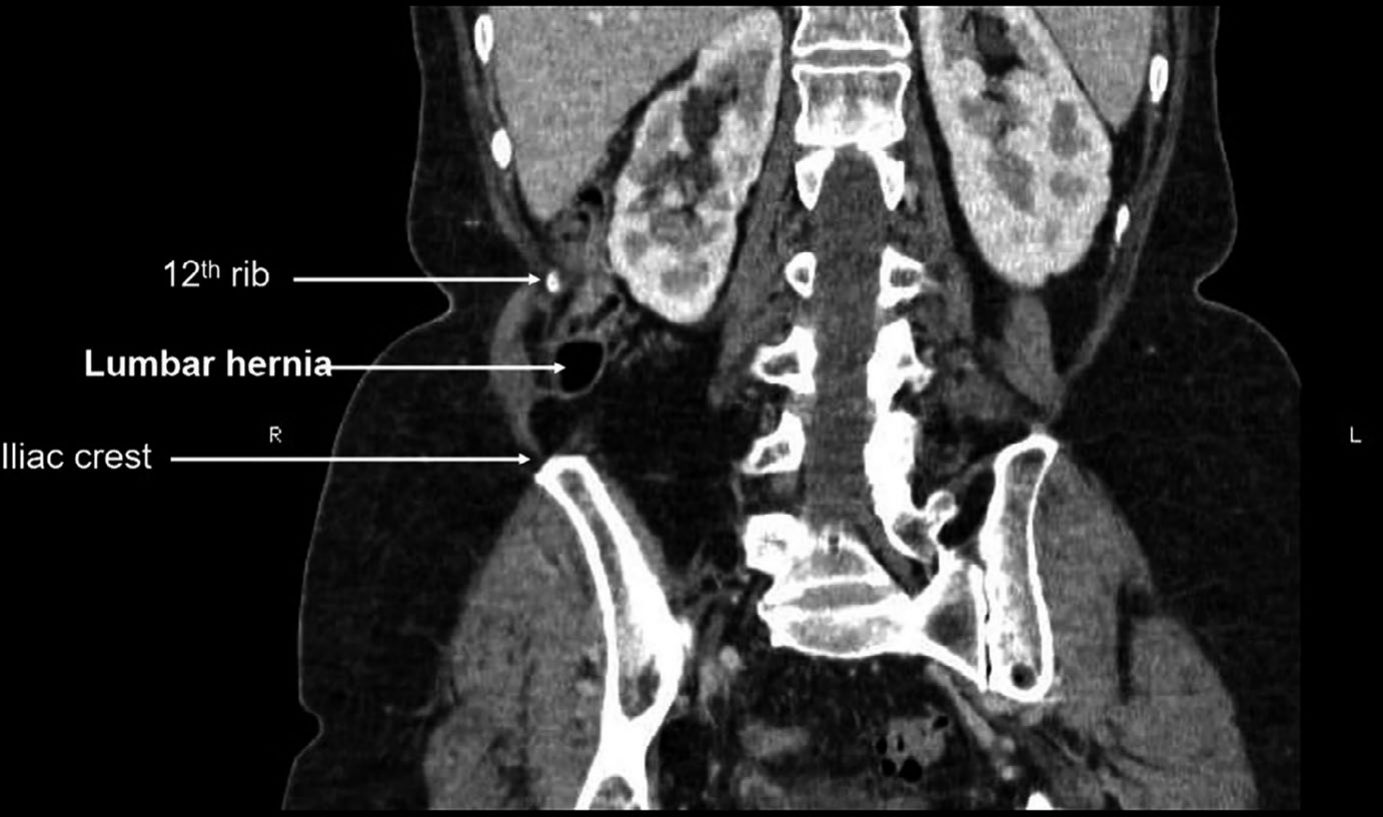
Coronal slice of CT abdomen showing area of hernia relative to 12th rib and iliac crest
